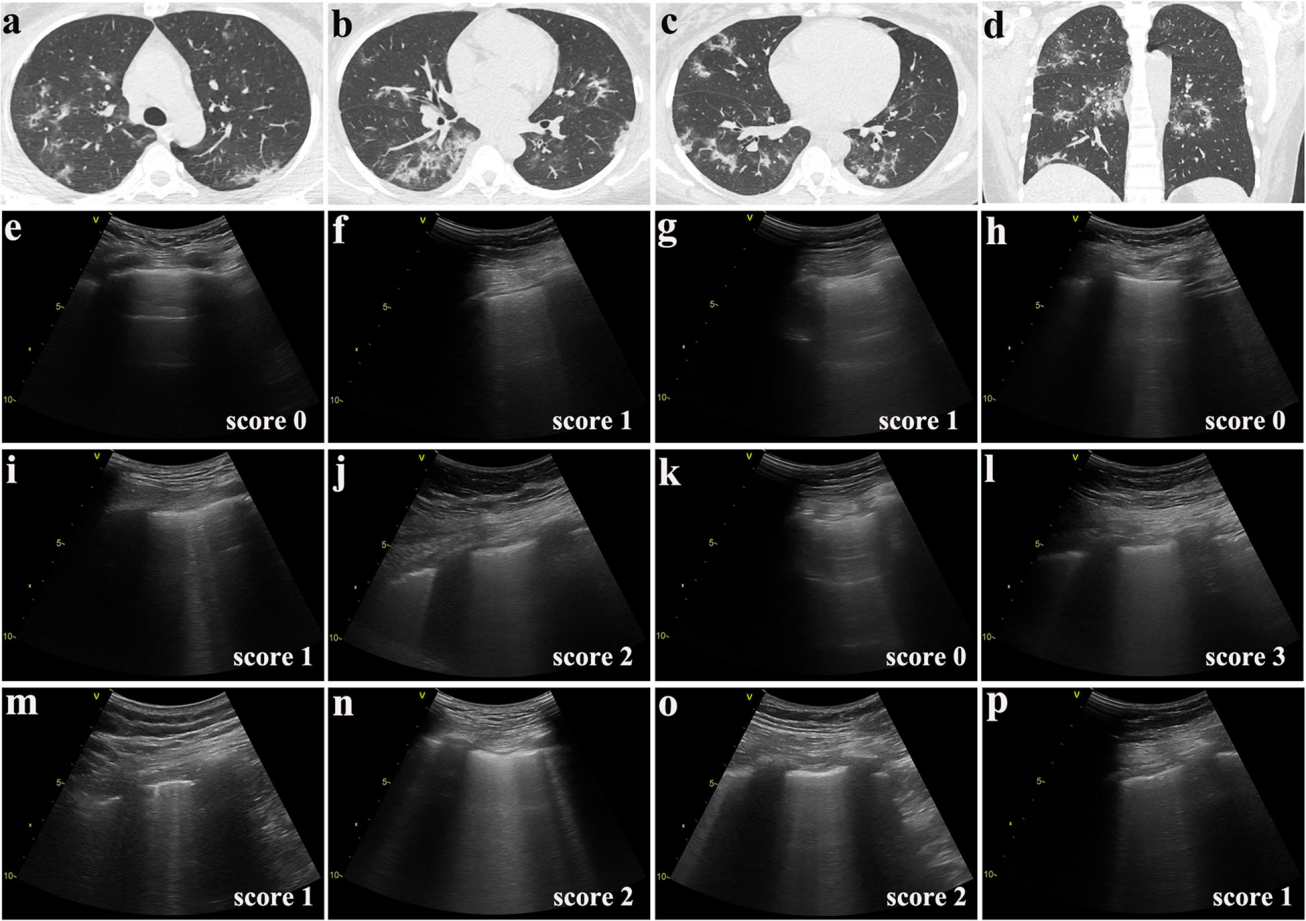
Chest CT and lung ultrasound imaging of a typical patient. a-d Chest CT showed involvement of all five lobes of the bilateral lungs in this patient, and the total CT score was 10 points. e-p The lung ultrasound findings in 12 zones. e: right anterior upper zone, f: right anterior lower zone, g: left anterior upper zone, h: left anterior lower zone, i: right lateral upper zone, j: right lateral lower zone, k: left lateral upper zone, l: left lateral lower zone, m: right posterior upper zone, n: right posterior lower zone, o: left posterior upper zone, p: left posterior lower zone. The total ultrasound score was 14 points
